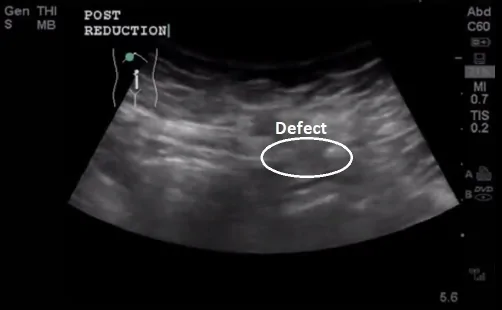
Following the use of ultrasound to identify the abdominal wall defect and gently apply pressure to the herniated bowel, the hernia reduction was confirmed using ultrasound. While the defect in the abdominal muscles can still be seen, it is now free of bowel.
